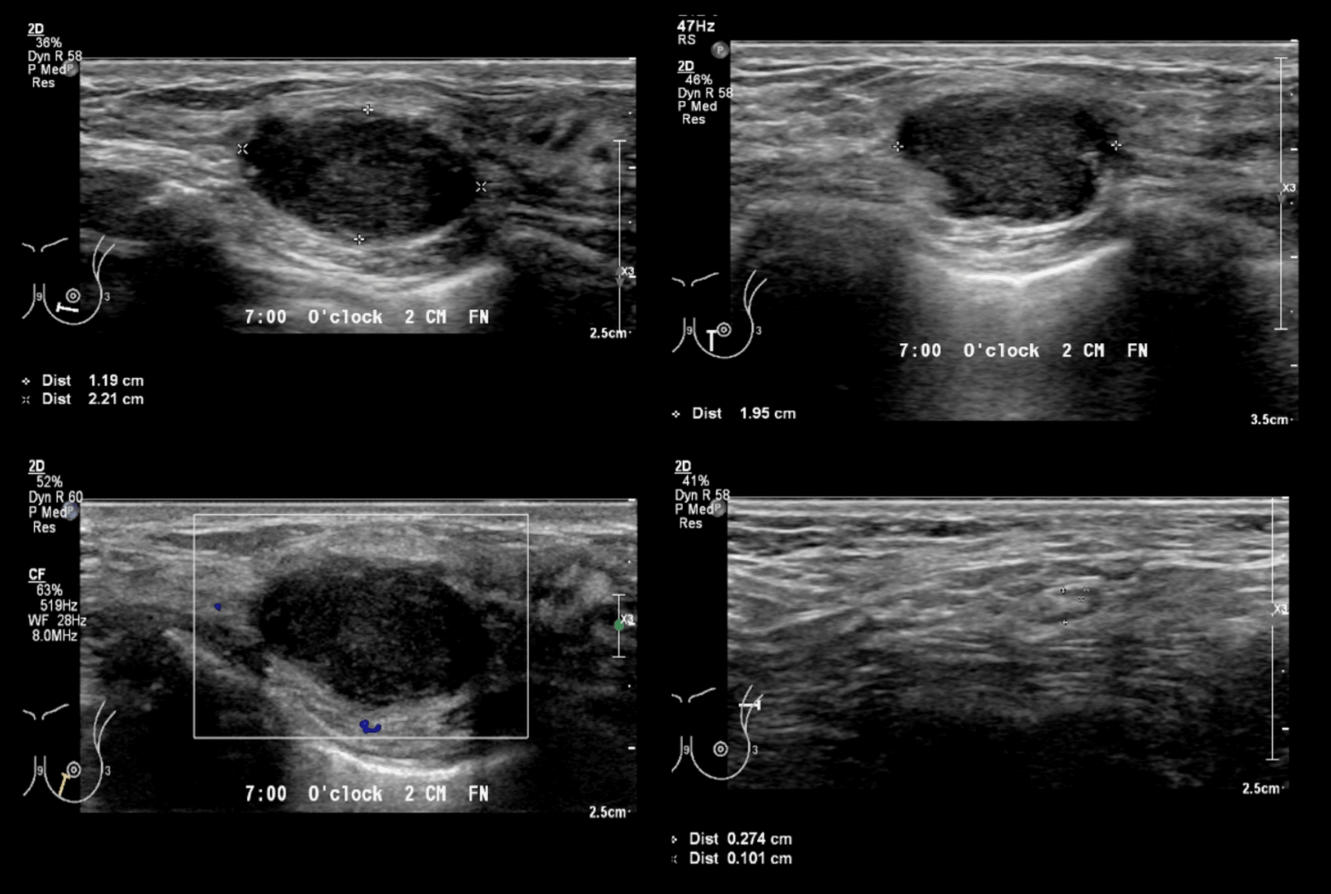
Left breast ultrasound showing micro-lobulated hypoechoic lesion at 7 o’clock 2 cm FN (1.2x2.2x2.0 cm). No internal vascularity, posterior features, or significant lymphadenopathy on the left. FN: From nipple
